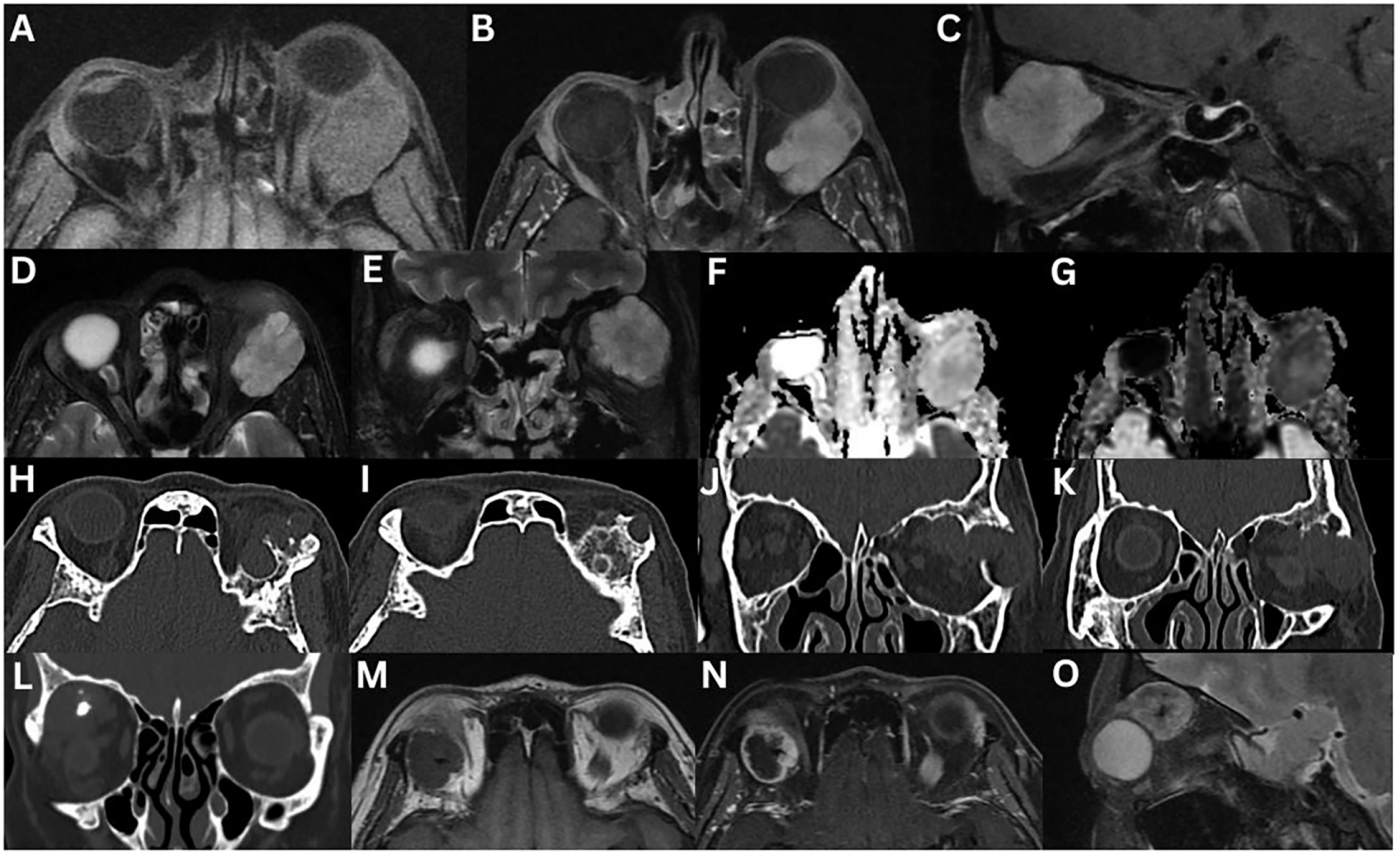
Pleomorphic adenoma of the left lacrimal gland (A–G). T1-weighted axial FLAIR fat-suppressed (A), T1-weighted fat-suppressed contrast-enhanced axial (B) and sagittal (C), T2-weighted axial (D), T2-weighted fat-suppress contrast-enhanced coronal (E) sequences are demonstrated. A large, irregular, lobulated mass occupies the lacrimal gland. The lesion appears heterogeneous with intermediate signal intensity and shows marked enhancement after contrast administration. There is significant compression and displacement of the lateral rectus muscle in the extraconal space, partially extending intraconally. There is no restricted diffusion on ADC (F) and eADC (G) sequences. In an 80-year-old female with a pleomorphic adenoma, CT axial (H, I) and coronal (J, K) views demonstrate the left lacrimal gland mass with bone formation and destruction. In a 55-year-old female with pleomorphic adenoma, coronal CT (L) demonstrates intralesional calcifications with no evidence of bony changes. T1-weighted axial (M), T1-weighted fat-suppressed contrast-enhanced axial (N), and T2-weighted sagittal fat-suppressed (O) sequences demonstrate an irregularly bordered, heterogeneous mass with intralesional hypointensity with could reflect cystic degeneration, mesenchymal stroma, necrosis, haemorrhage, or mucous/serous fluid collections. There is moderate enhancement following contrast administration. The mass demonstrates a low signal intensity on T1-weighted imaging, and an intermediate signal intensity on T2-weighted imaging.
